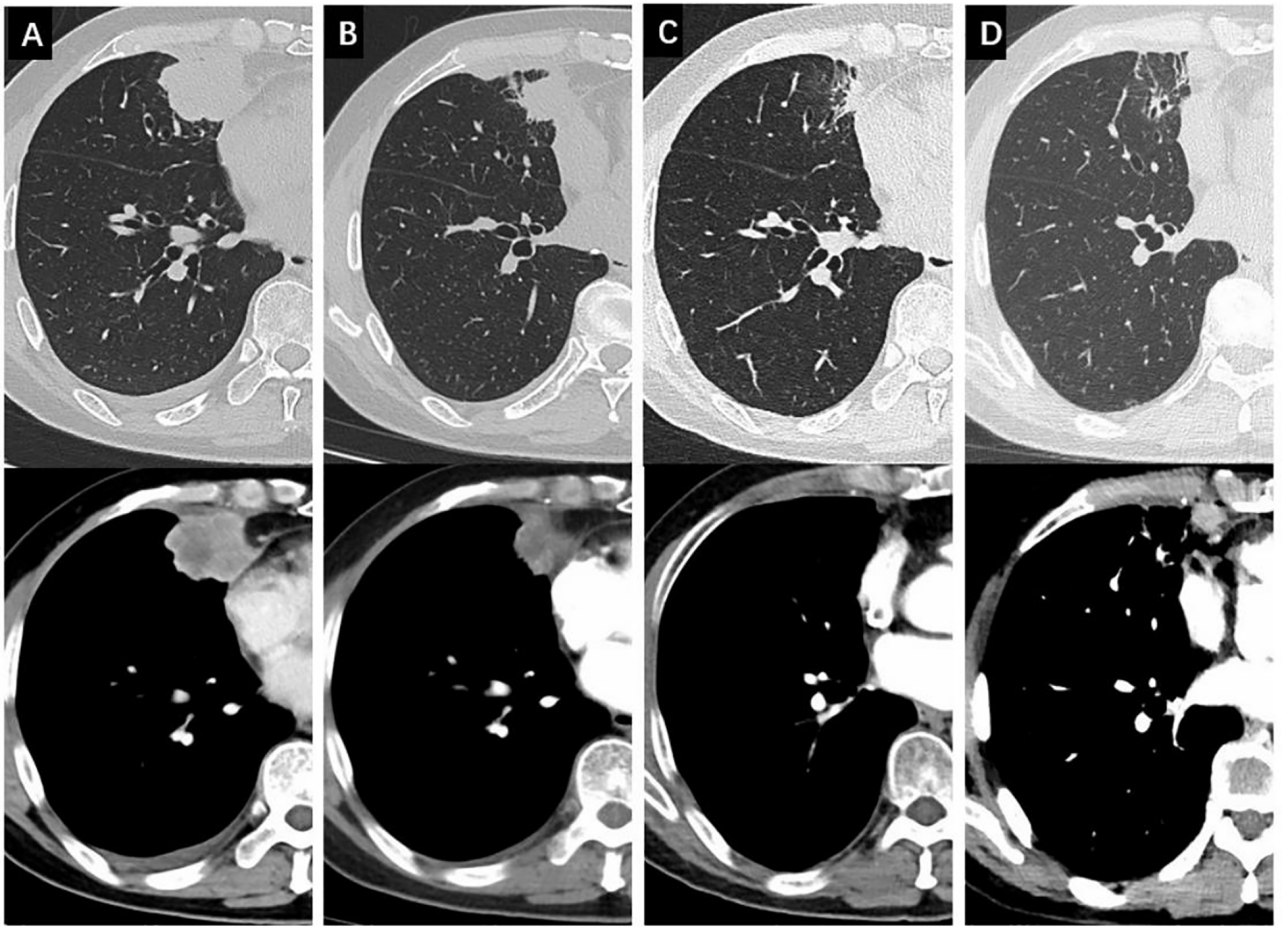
Changes of the non-cavitated SqCLC in the right middle lobe in both the lung window and the mediastinal window. (A) baseline CT before osimertinib treatment; (B) changes of the primary lesion after 1 months of osimertinib; (C) changes of the primary lesion after 14 months of osimertinib; (D) changes of the primary lesion after 16 months of osimertinib with a newly emerged lesion in the right diaphragmatic angle.
